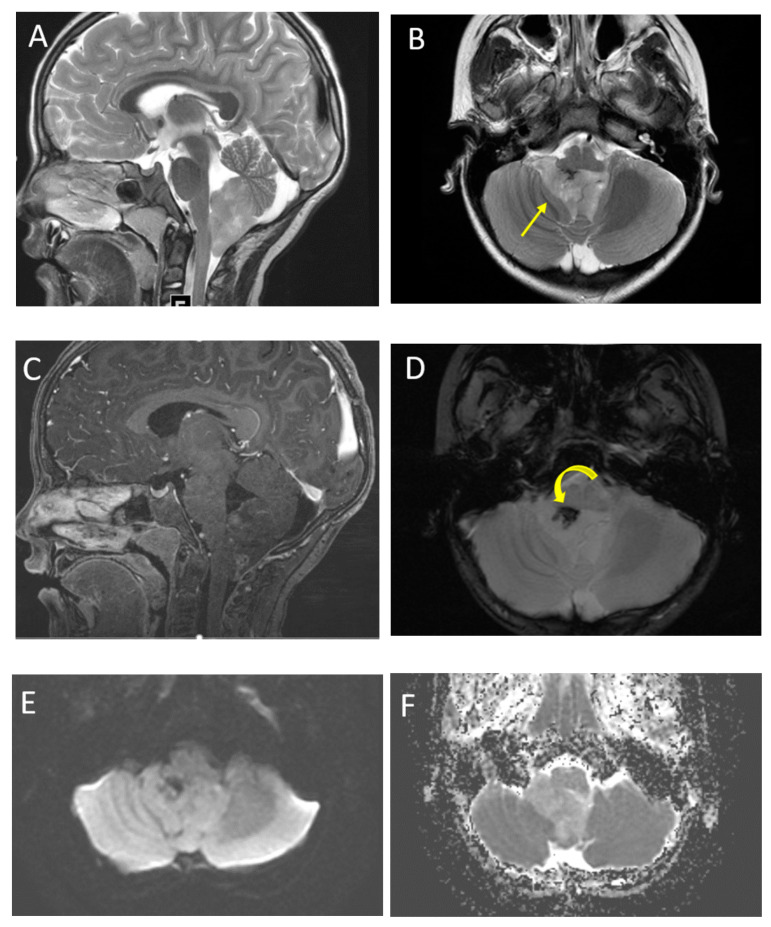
A 6-year-old male with ependymoma. Sagittal and axial T2 images (A,B) demonstrate a heterogeneous lesion involving the fourth ventricle. Note the characteristic extension through the foramen of Luska (arrow). Post-contrast T1 imaging (C) demonstrates typical heterogeneous enhancement. SWI imaging (D) demonstrates a focus of low signal correlating with calcification (curved arrow). DWI (E) and ADC (F) imaging show increased ADC values of the tumor compared with adjacent parenchyma.
